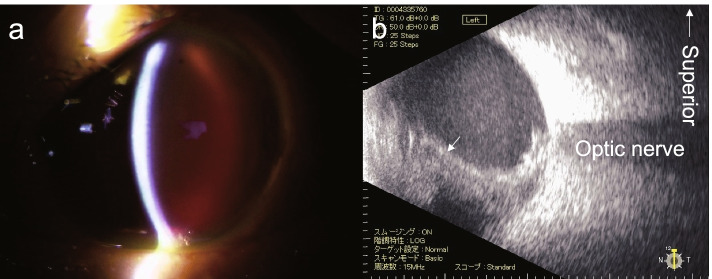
Intraocular hemorrhage after the initial surgery. Bleeding in the anterior chamber (a). Hyperechoic image of vitreous hemorrhage during ocular ultrasound examination (b, arrow)
